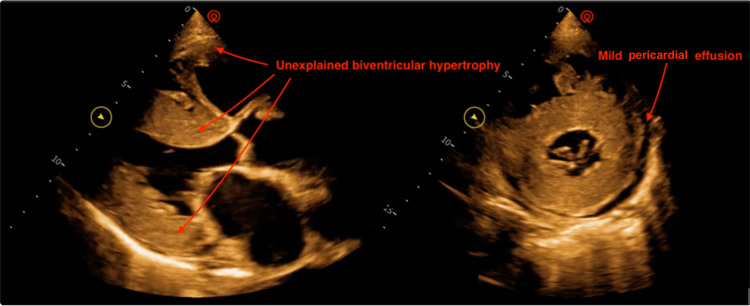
Transthoracic echocardiography showing unexplained biventricular hypertrophy with mild pericardial effusion and mildly thickened valve leaflets
